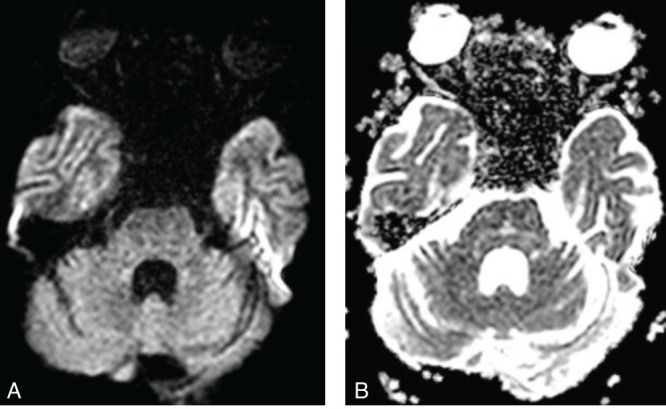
Axial DWI (A) and corresponding ADC (B) images showing no high signal intensity on the DWI image, with corresponding high signal on the ADC map.
